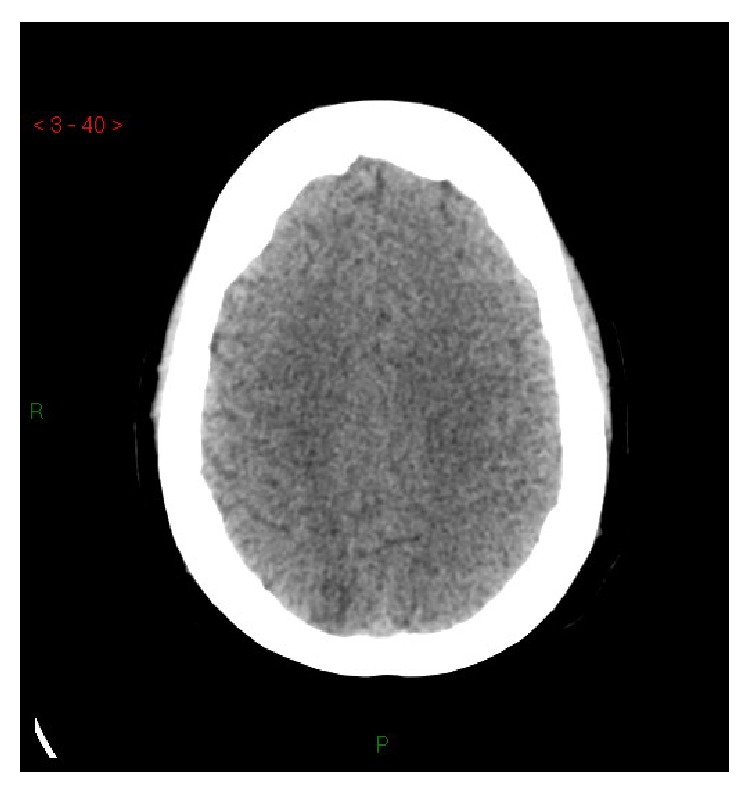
Case 2. Basal CT: hypodense cortical-subcortical lesions of the medial parietal region.
